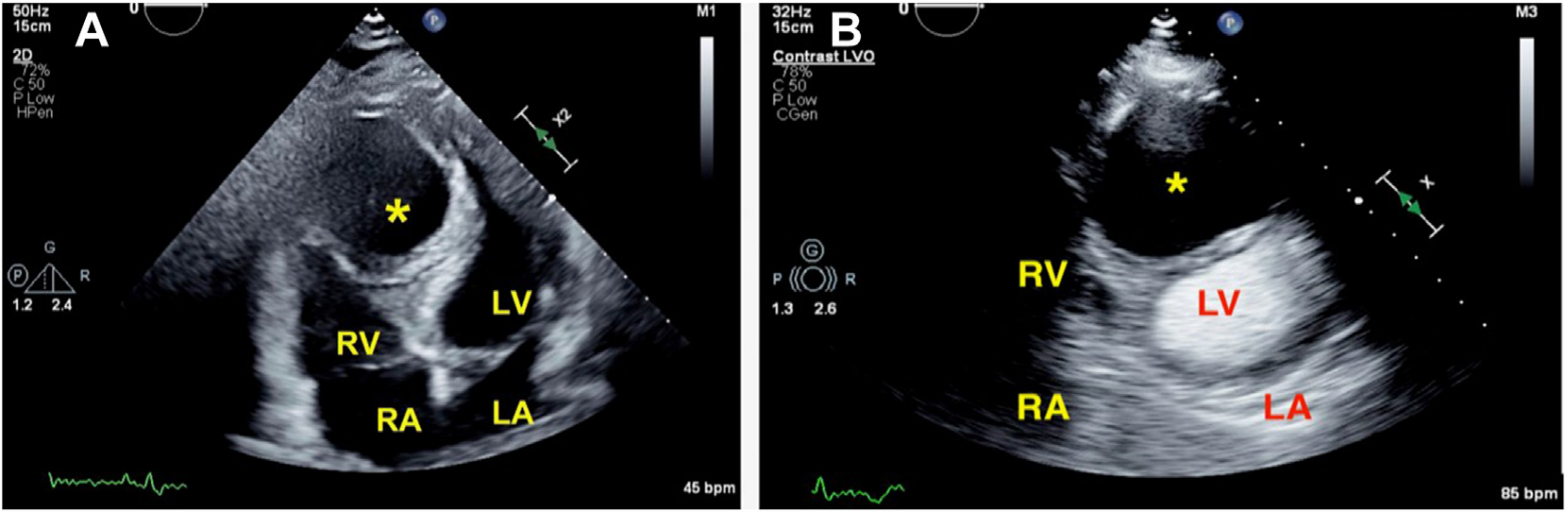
Two-dimensional TTE, apical four-chamber view, systolic phase before (A) and after (B) the administration of an ultrasound-enhancing agent, demonstrating a large echo-free (cystic) mass (*asterisk*) in the middistal IVS.
